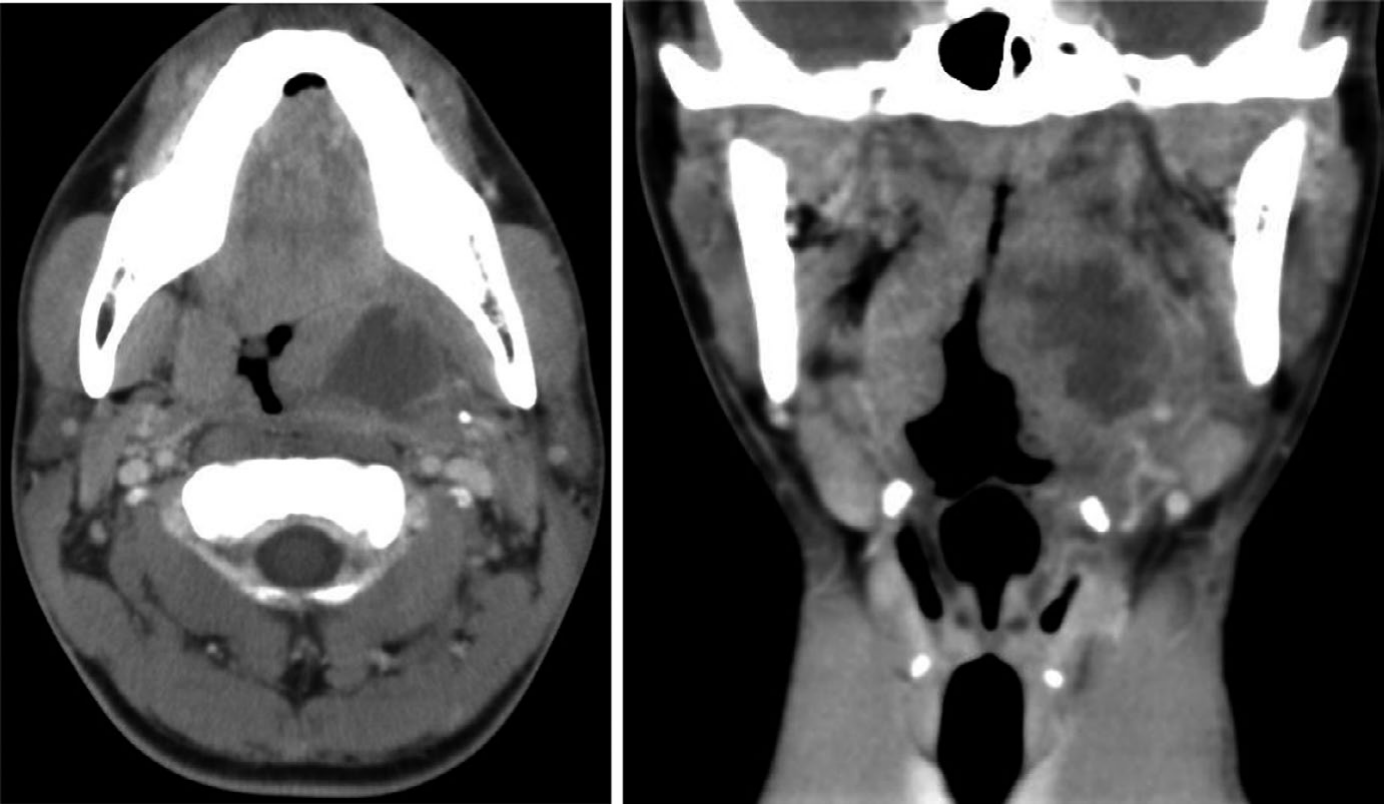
Contrast‐enhanced computed tomography (CT) of the lateral side of the left tonsil revealed a low‐density margin of contrast enhancement measuring 26 mm × 22 mm
